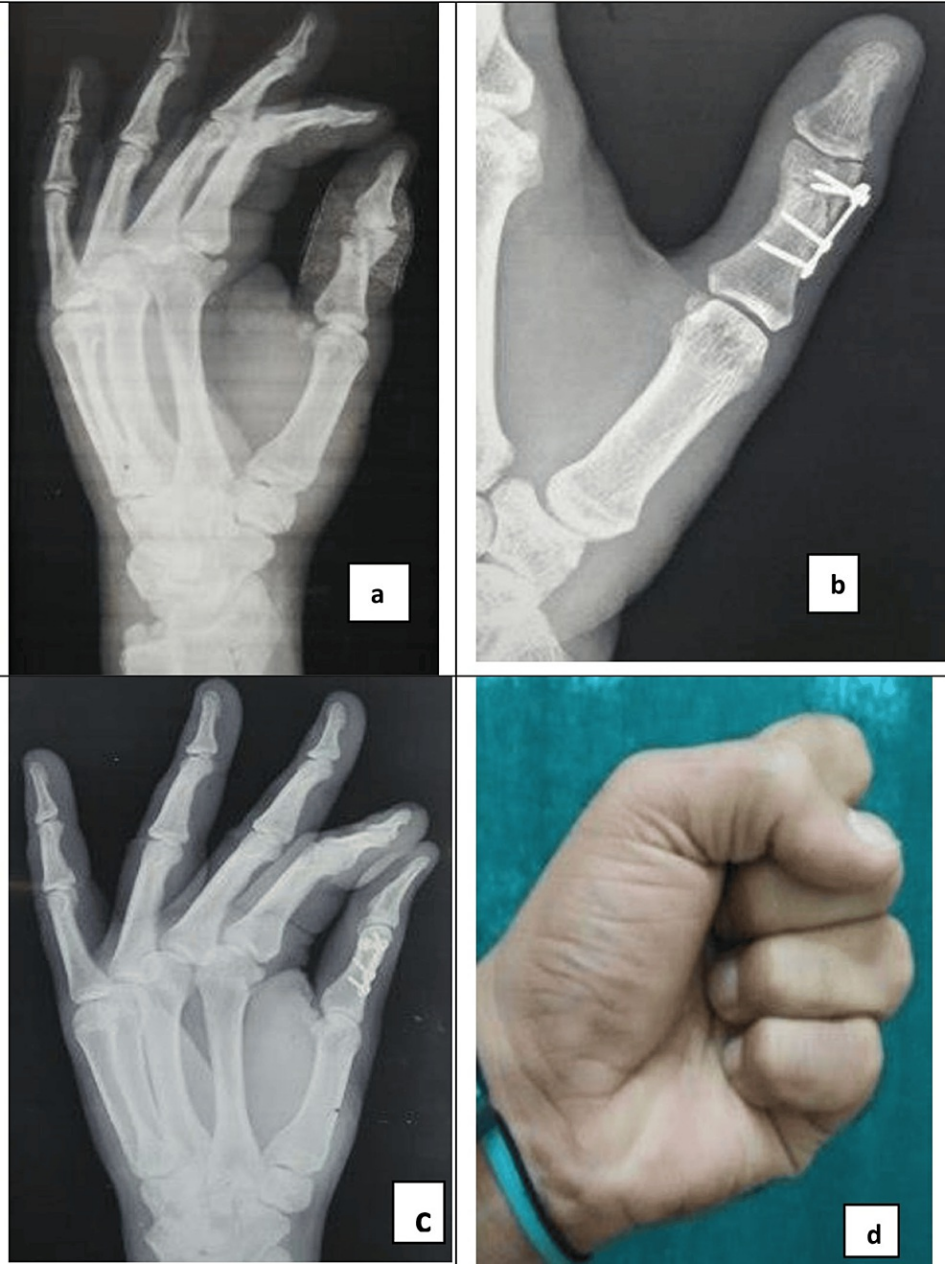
Radiograph showing Intra-articular fracture of 1st proximal phalanx (a) and after open reduction and fixation with a T plate (b). Radiograph showing complete union at fracture site (c), and clinical picture showing excellent active range of motion (d).
